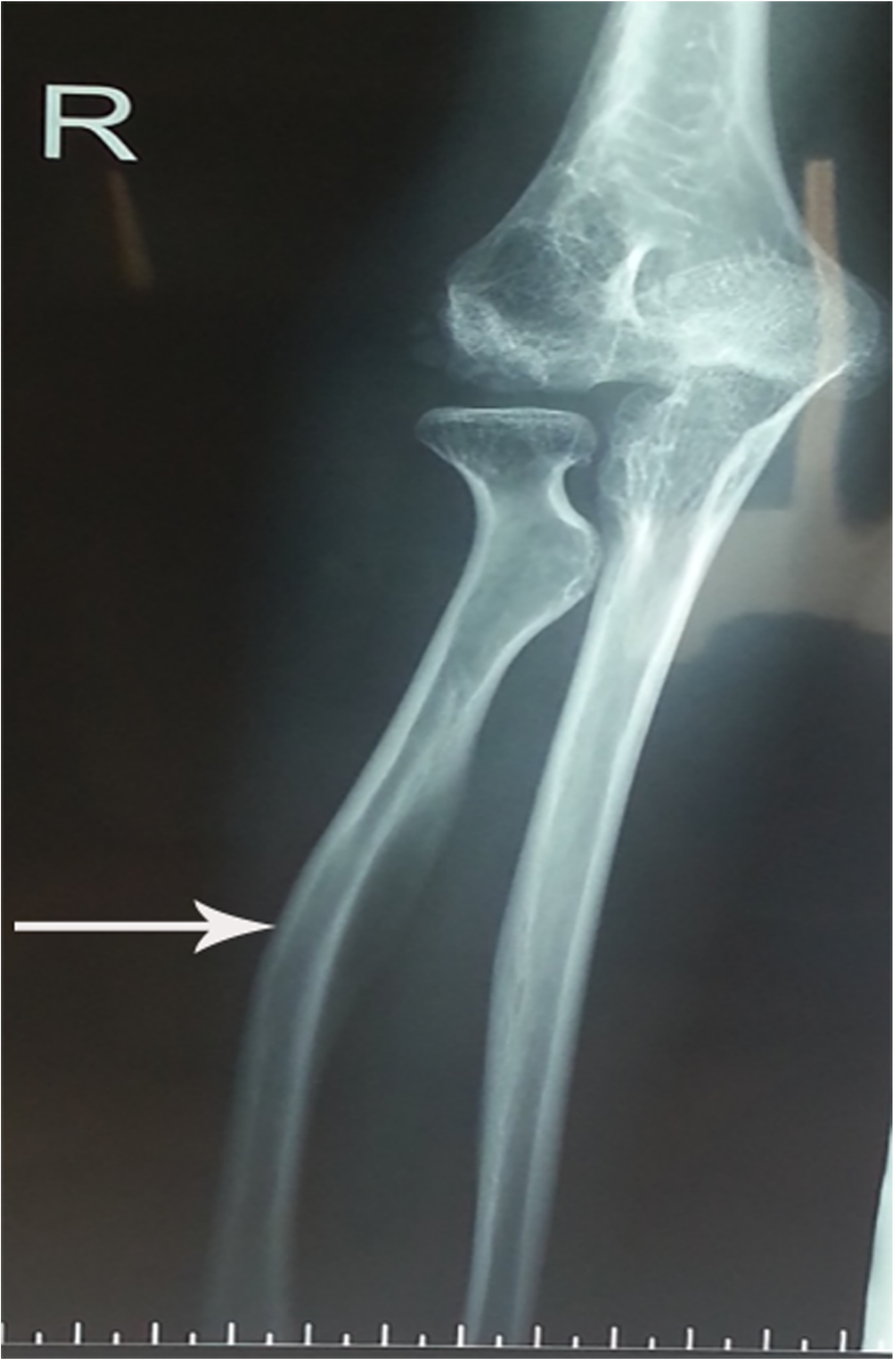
The X-ray radiograph of the 2^nd^ patient shows that the distal radius was tilted towards the ulna
